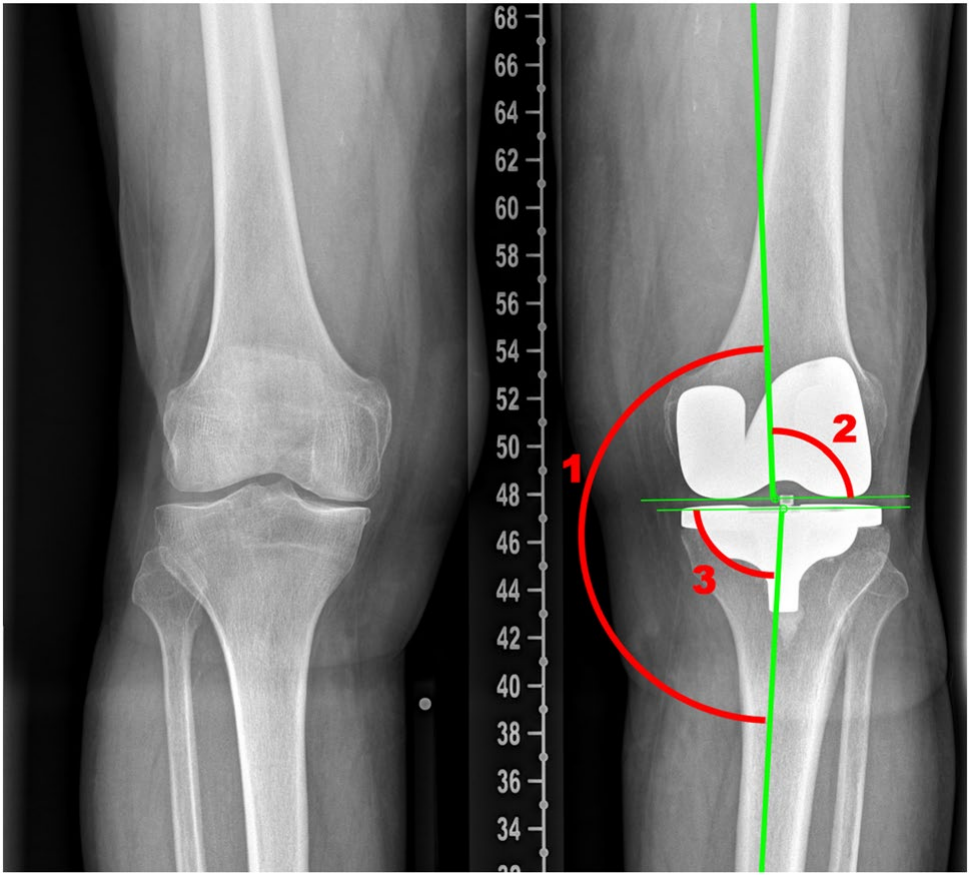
Measured angles on anterior–posterior view X-ray—HKA (1), LDFA (2) and MPFA (3)
